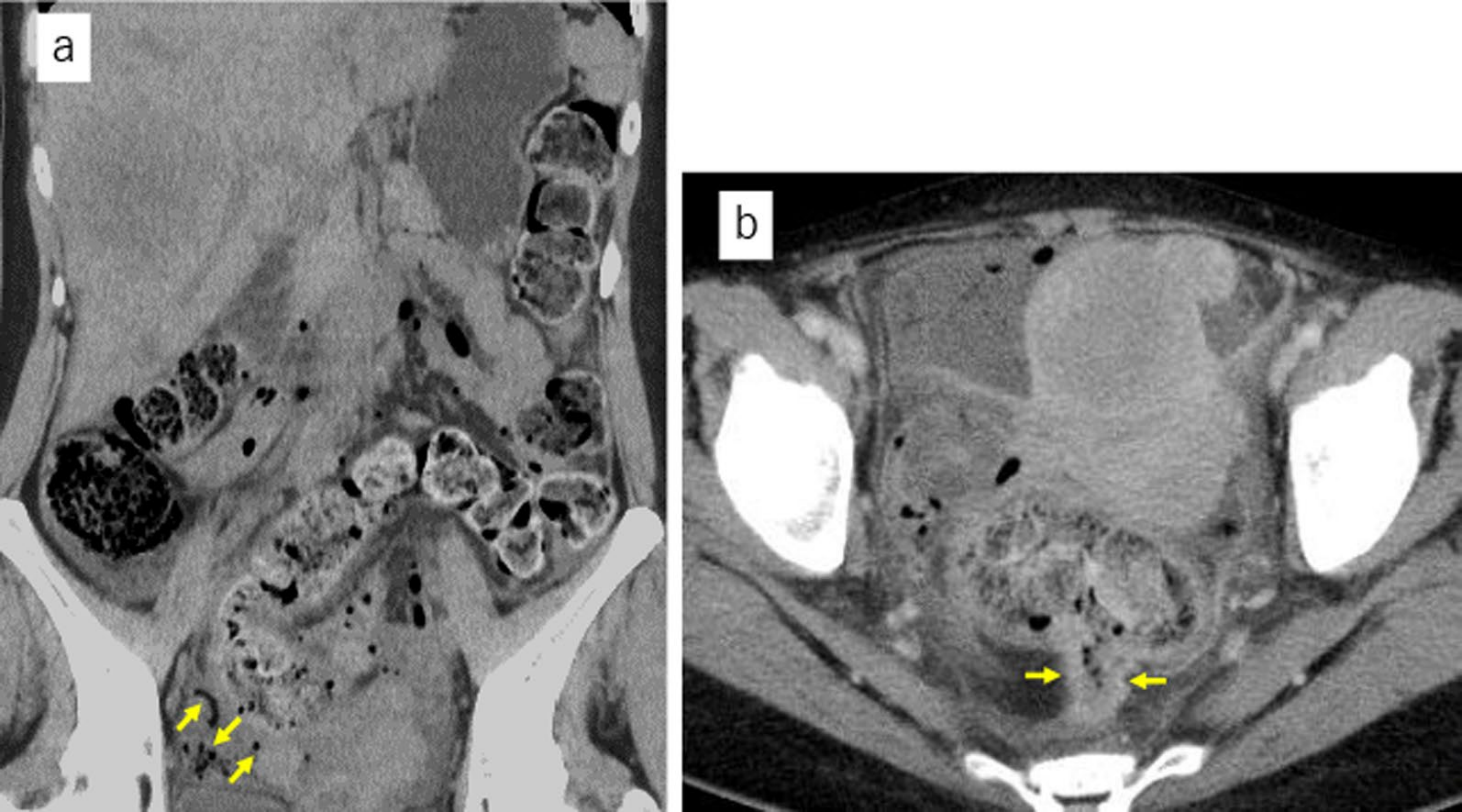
Computed tomography in Case 1. a Computed tomography showed a large amount of fecal mass in the colon and free air (yellow arrows) in the pelvis. A large mass was observed in the liver. b There was wall thickening in the rectum (yellow arrows), and the oral side of the rectum was dilated and had a fecal mass. Multiple uterine fibroids were seen, and ascites with free air was observed around them
